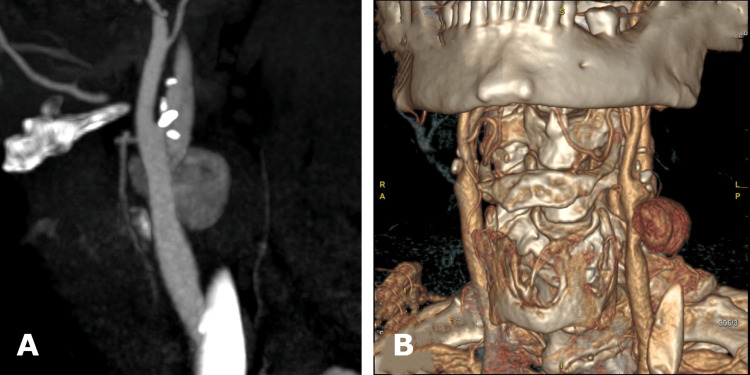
CTA revealing a 28 × 20 mm pseudoaneurysm of the left common carotid artery (CCA), located approximately 15 mm proximal to the carotid bifurcation, without evidence of active extravasation. A) 2D sagittal image of the pseudoaneurysm; B) 3D reconstruction of the pseudoaneurysm.
